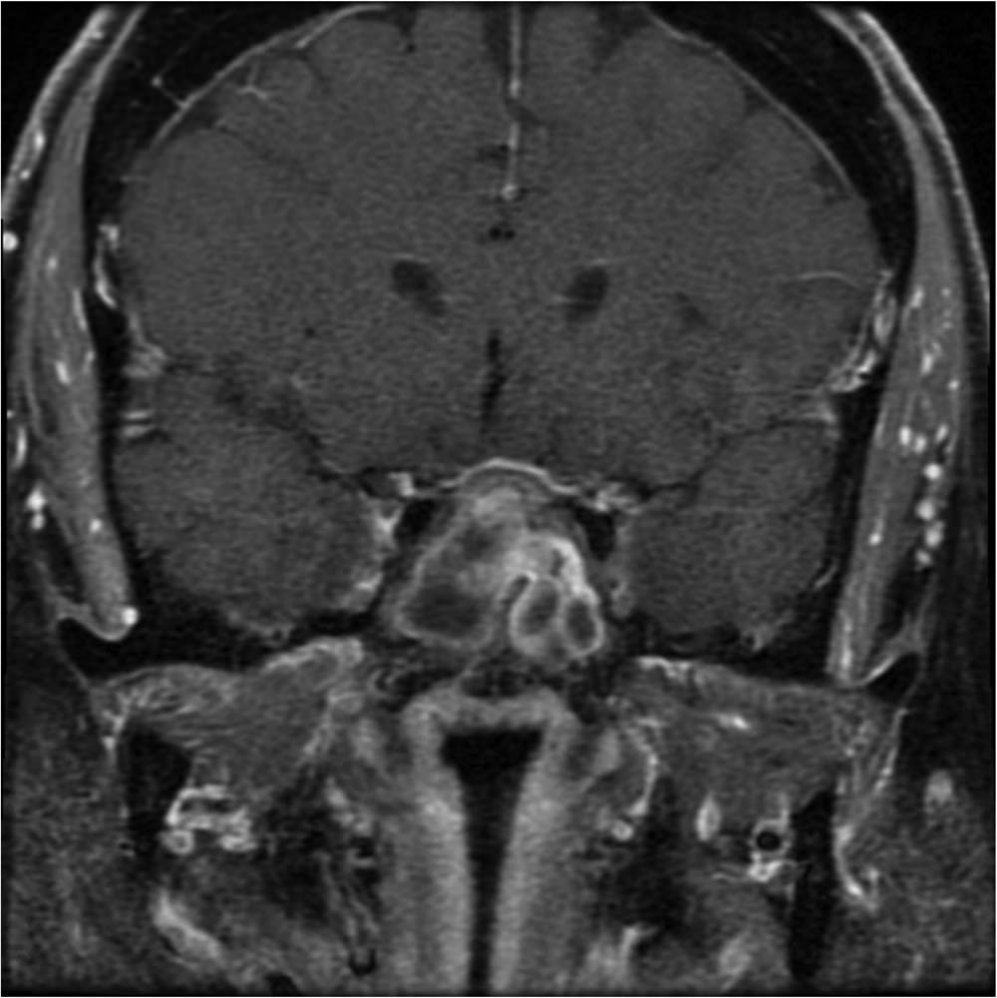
A coronal brain MRI showing a heterogeneous, enhancing lesion located within the sphenoid sinus, extending superiorly to the sellar and parasellar regions. The mass shows irregular borders and appears to cause a mild compression of the optic chiasm.
